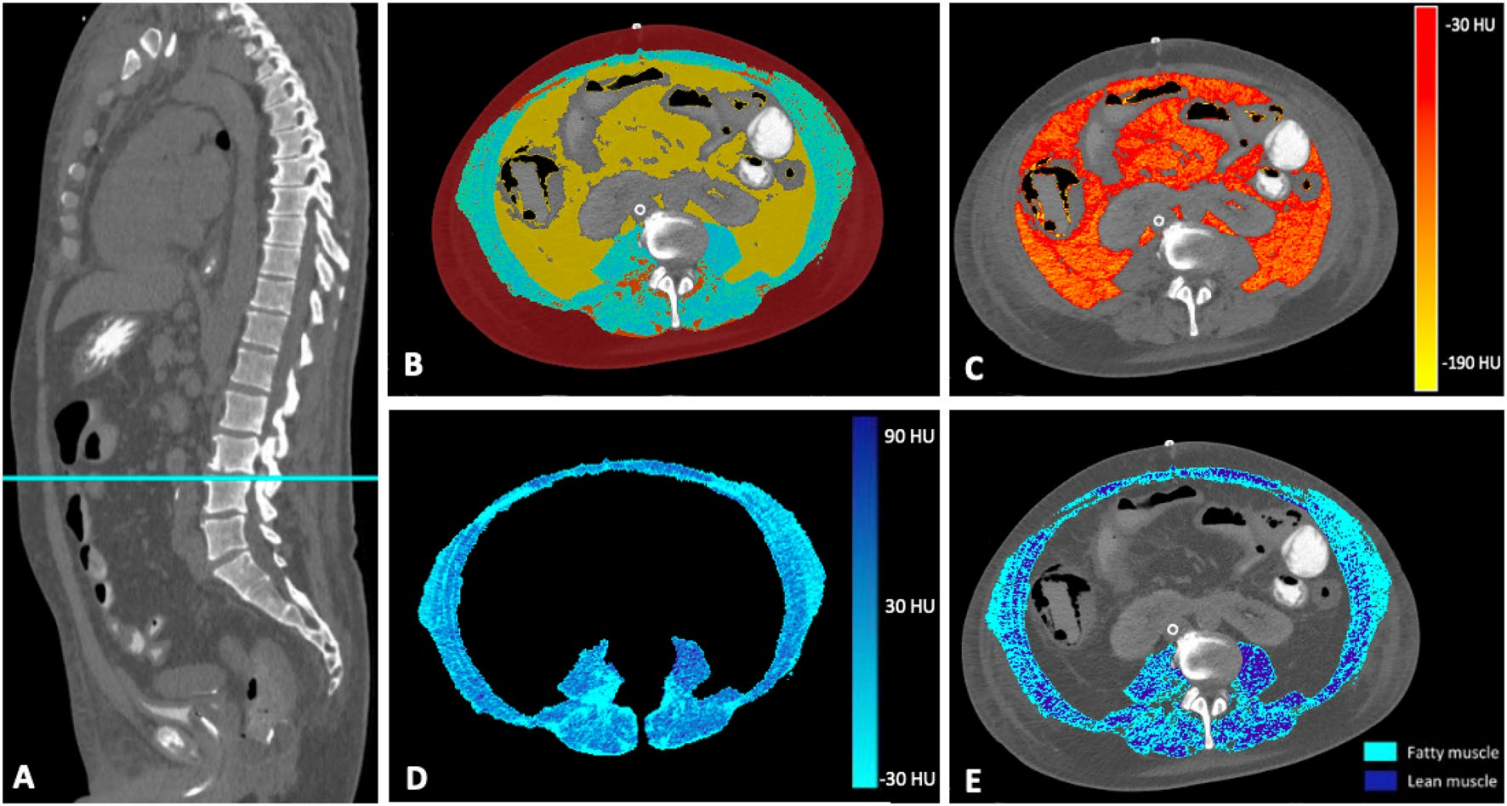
Synopsis of body composition analysis. Single-slice cross sectional CT images at the level of the intervertebral disc space L3/L4 were exported for analysis (A). Areas of visceral adipose tissue (yellow) were separated from subcutaneous adipose tissue (red) and abdominal skeletal muscle (blue) and assessed within these cross-sections based on densitometric thresholds (B). In addition to adipose tissue area, mean attenuation of visceral adipose tissue area was calculated to determine overall inflammatory activity (C). Mean muscle attenuation of the entire skeletal muscle area was calculated to obtain overall myosteatosis (D). Muscular fat infiltration was also quantified by separating the skeletal muscle area into areas of fatty and lean muscle using attenuation thresholds of − 30 to 29 Hounsfield units (HU) and 30 to 100 HU, respectively (E). CT = computed tomography, HU = Hounsfield Units.
